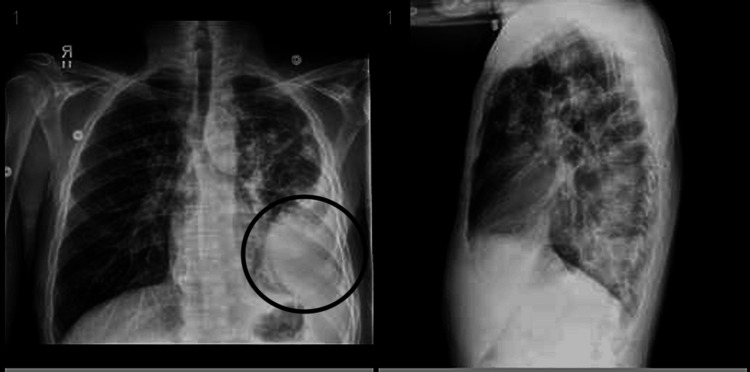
Chest X-ray on admission, showing a large opaque density projecting over the left lower hemithorax.
